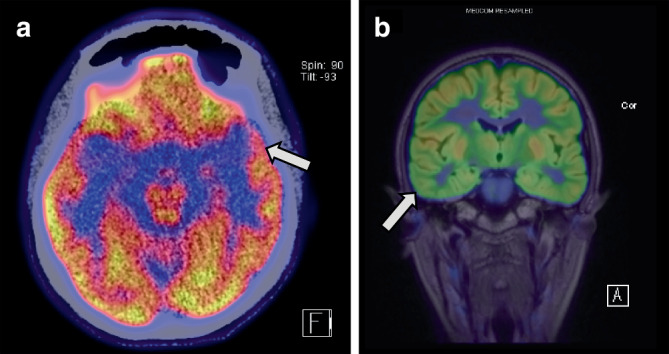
a FDG-PET/CT of participant 1, axial view. Statistical comparison demonstrated hypometabolism in the left temporal pole (−12%, *arrow*), left orbitofrontal cortex (−9%) and left insula (−5%). b FDG-PET/MRI of participant 7 demonstrating focal hypometabolism of the right lateral inferior and middle temporal gyrus (*arrow*), coronal view
